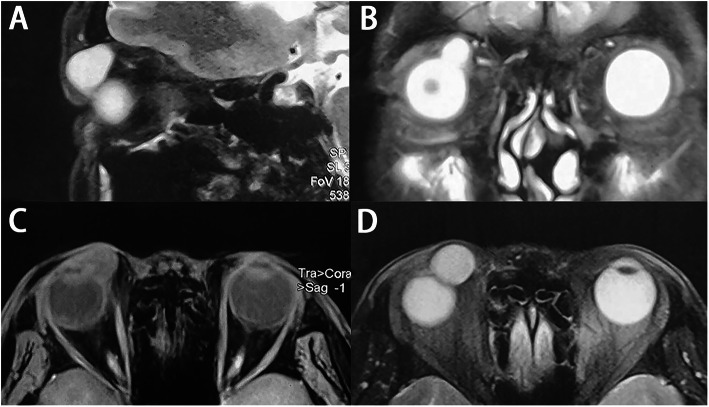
Magnetic resonance imaging shows a fluid-density cyst in the right superior medial orbit. a, Sagittal plane, b, Coronal plane, c, Axial plane T1weighted, d, Axial plane T2weighted
